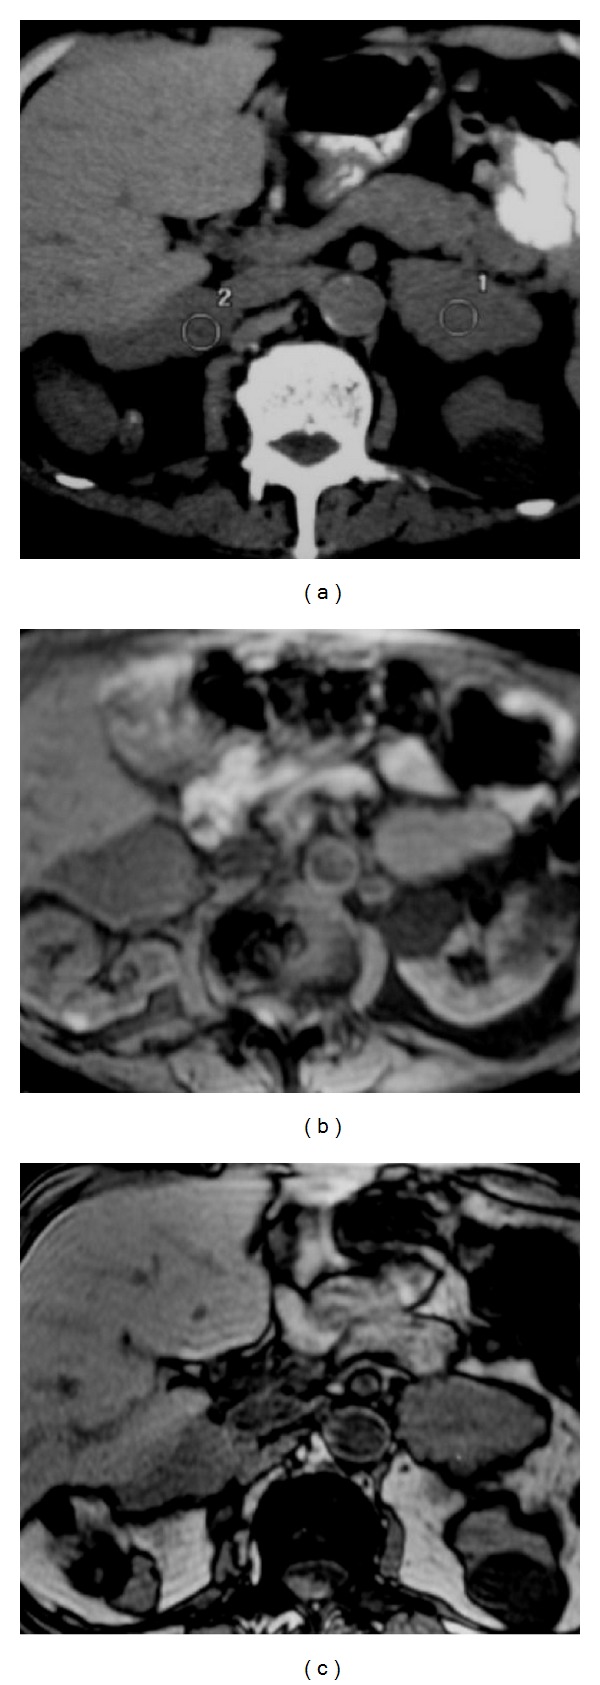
Adrenal lymphoma. Axial unenhanced CT (a) shows irregular large bilateral adrenal glands. MR axial images (b) in phase and (c) out of phase demonstrate enlarged adrenal glands.
